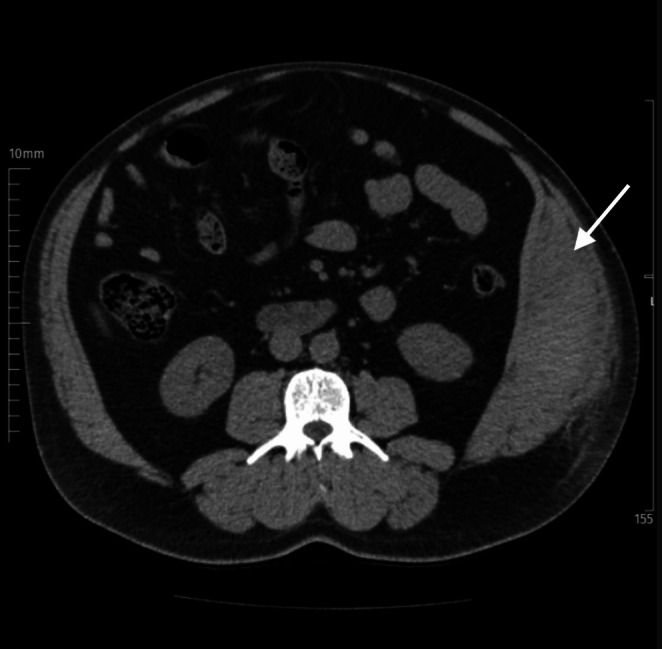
Computed tomography of the abdomen showing a 10.5 × 6.5 × 5.5 cm hematoma in the left internal oblique muscle (arrow).
